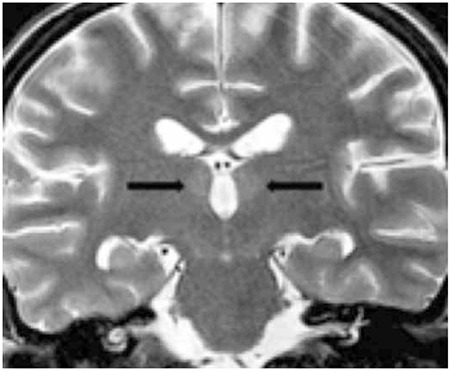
Coronal T2-weighted image shows symmetrical, weak, and limited increased signal intensity in the medial thalami and in the third ventricle-facing surface.
